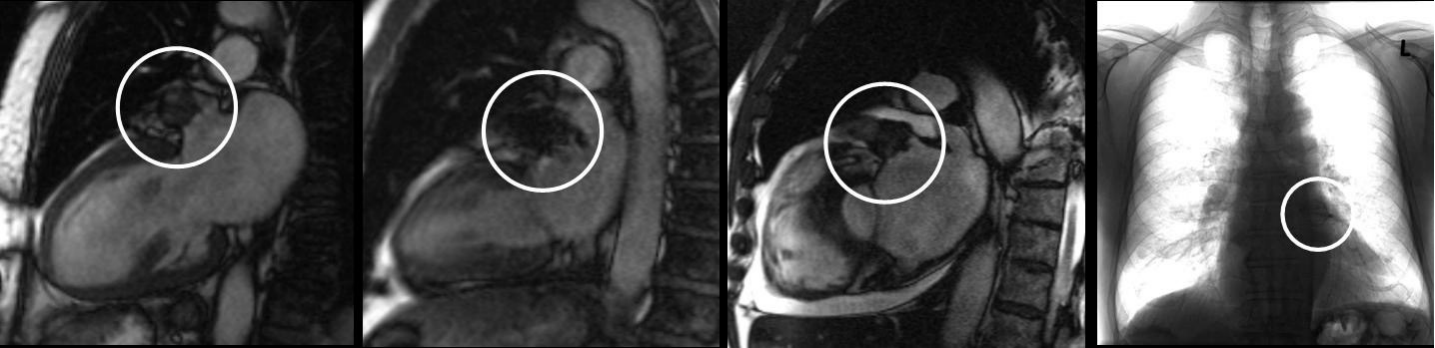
Different appearance of LAA devices. Cine TrueFISP-SSFP sequences demonstrate signal voids within LAA representing the correct placement and the exclusion of a migration of the devices: 1A (left panel) PLAATO device; 1B Watchmen device and 1C ACP device. For comparison figure 1D (right panel) shows a chest-X-ray of a PLAATO device.
